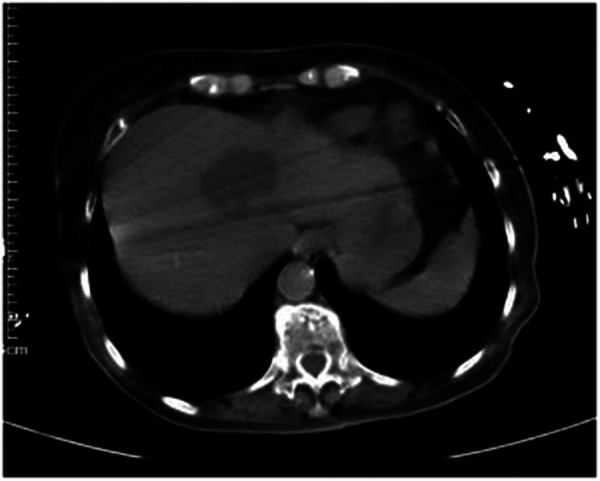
CT on admission (45 mm × 38 mm).
